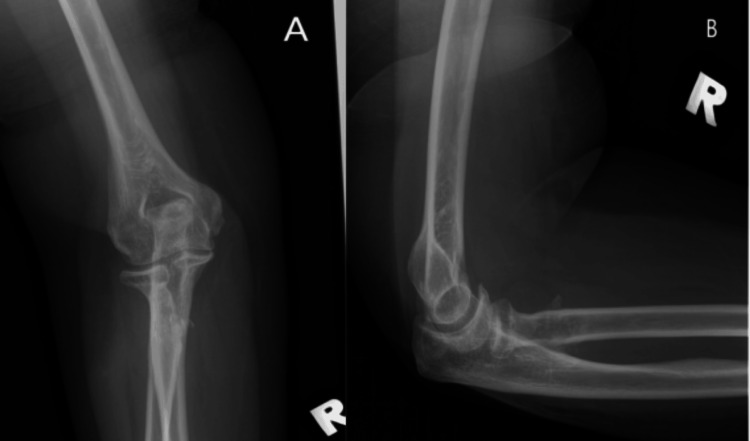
Right elbow radiograph AP view (A) and lateral view (B) four months post-excision showing no new ossification in the PRUJ interval AP: anteroposterior; PRUJ: proximal radioulnar joint
